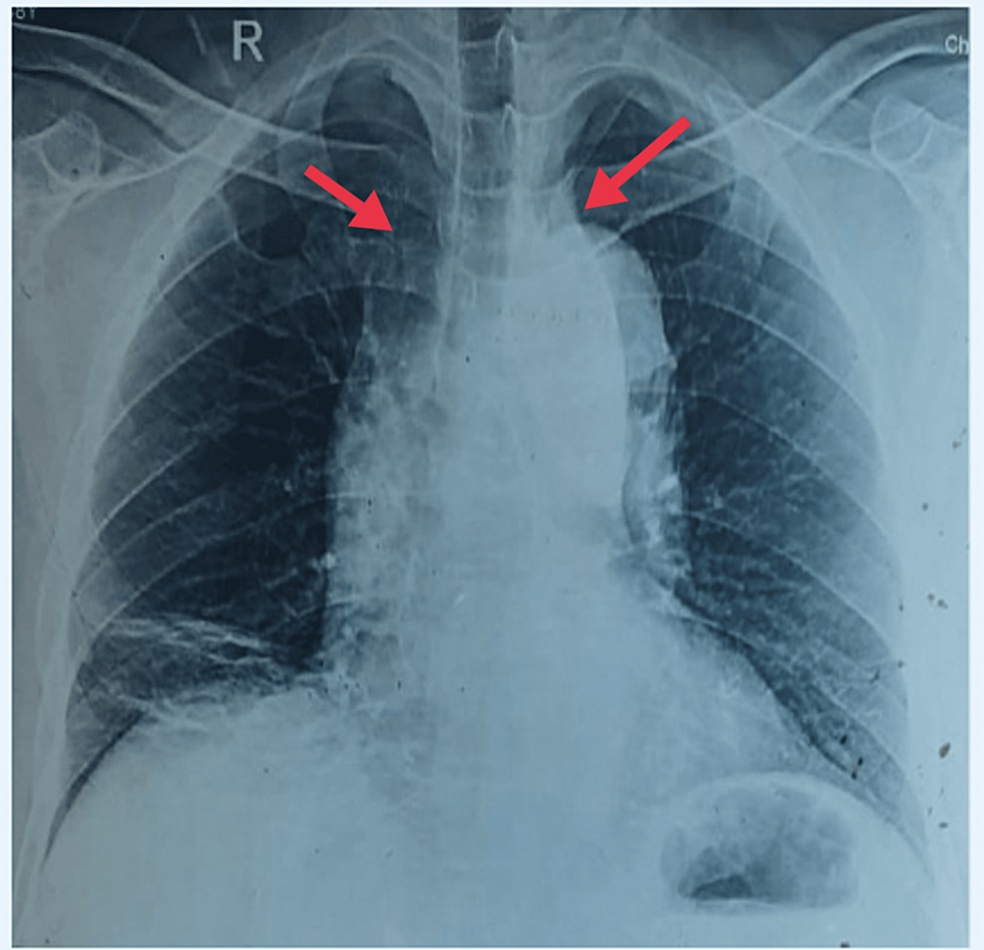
Chest X-ray showing superior widened mediastinum
